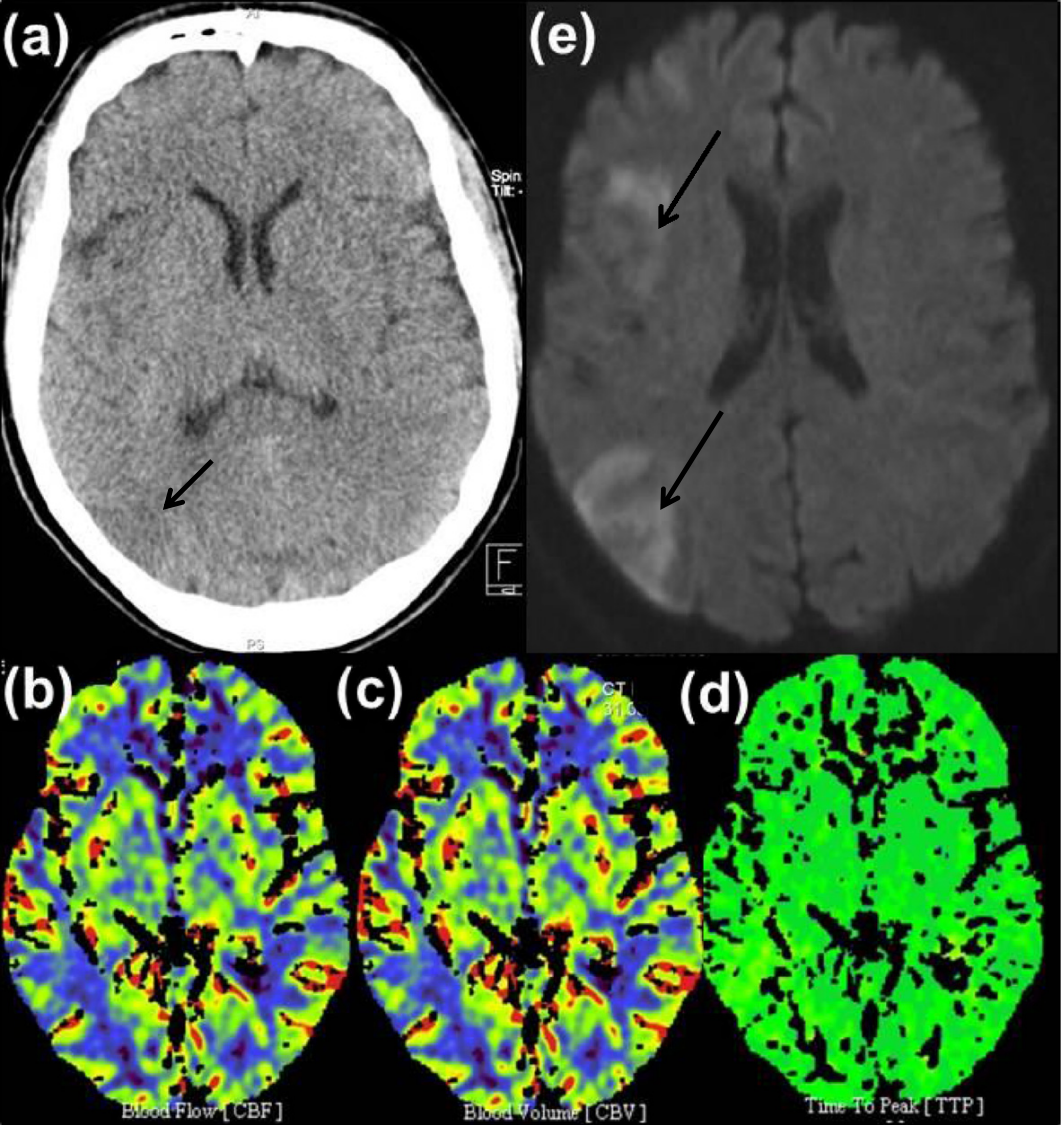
Native cranial computed tomography one day after treatment (a) and magnetic resonance imaging (diffusion-weighted imaging) 4 days later (e) display limited infarcted areas in right insula and watershed to posterior circulation. There is neither mismatch nor any perfusion deficit besides the above mentioned limited infarcted areas in the right middle cerebral artery territory in computed tomography perfusion imaging the day after intervention (b)-(d).
